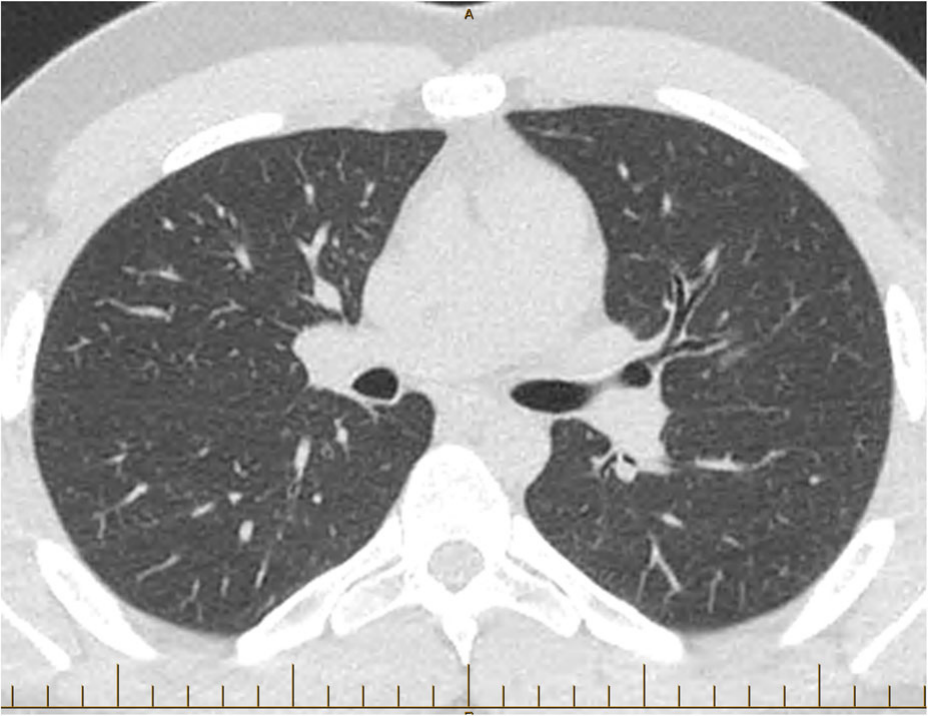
High‐resolution computed tomography (HRCT) scan of the patient at two years follow‐up demonstrating resolution of the prior changes.
